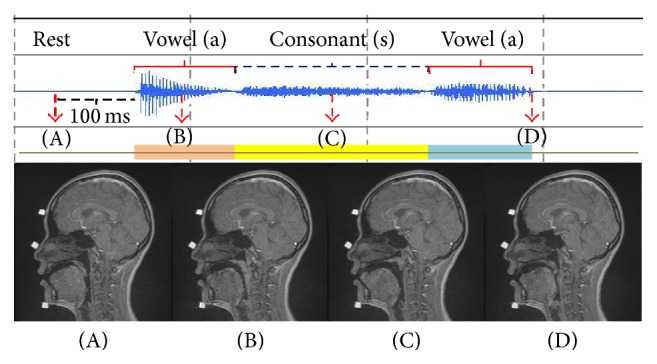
Representative sound data combined with movie MRI of a Japanese subject. A–D are the corresponding sound signals and MR images.
